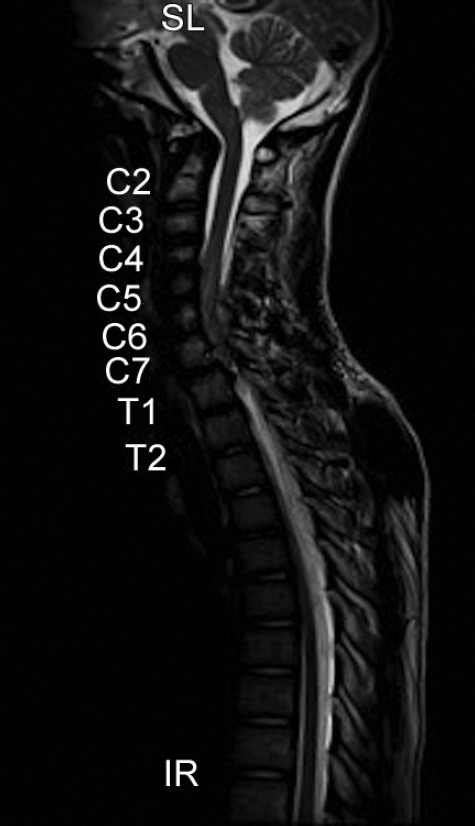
MRI spine and brain-extradural lesion of size 4.5 × 0.8 cm at C5-7 with cord compression and edema
